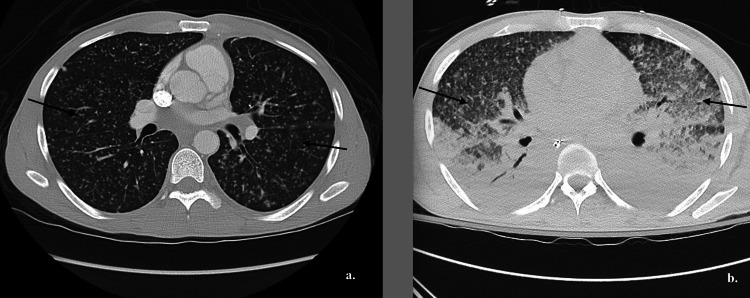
Evolution of CT chest. (a) CT chest on day 1, showing diffuse bilateral miliary nodules filling the entirety of both lungs, (b) CT chest on day 30, note the diffuse bilateral reticulonodular, flocculent, and consolidative airspace opacities.
